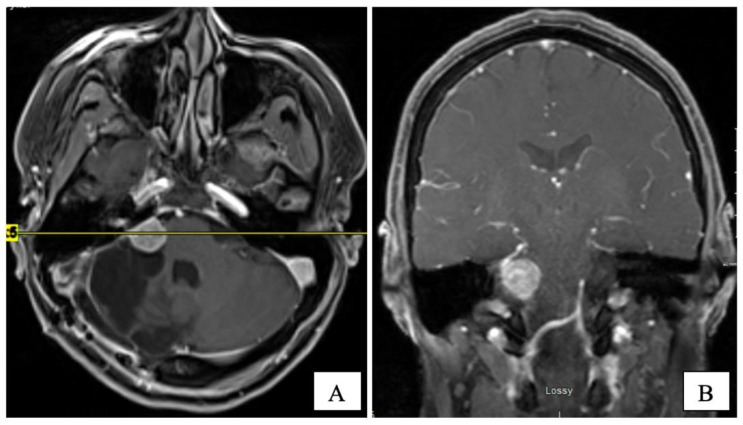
T1-W post-gadolinium MRI at 7 years post-op on axial (A) and coronal (B) planes. The CPA tumour measured 16 × 15 × 17 mm.
